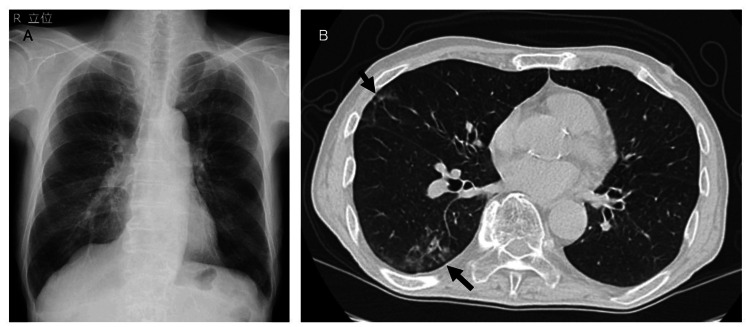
Chest X-rays and CT findings during the acute phase of COVID-19. (A) Chest X-rays showing no abnormalities during the acute phase of COVID-19, (B) while CT revealed mild ground-glass opacities in the right lung (arrows). COVID-19, coronavirus disease 2019; CT, computed tomography
